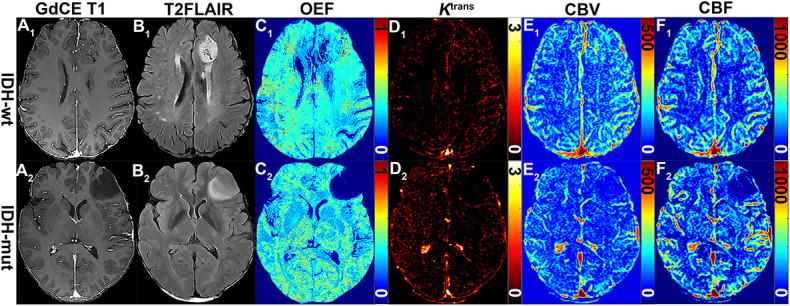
MRI-based OEF and DCE perfusion images in representative glioma patients with and without IDH mutation. Two exemplary patients with IDH1-wt (astrocytoma II; A_1_–F_1_) and IDH1-mut (astrocytoma II; A_2_–F_2_) lower-grade glioma shown: (A_1_) gadolinium contrast-enhanced (GdCE) 3DT1BRAVO, (B_1_) T2-FLAIR, (C_1_) QQ-based OEF maps by CAT, DCE-MRI with separated *K*^*trans*^
